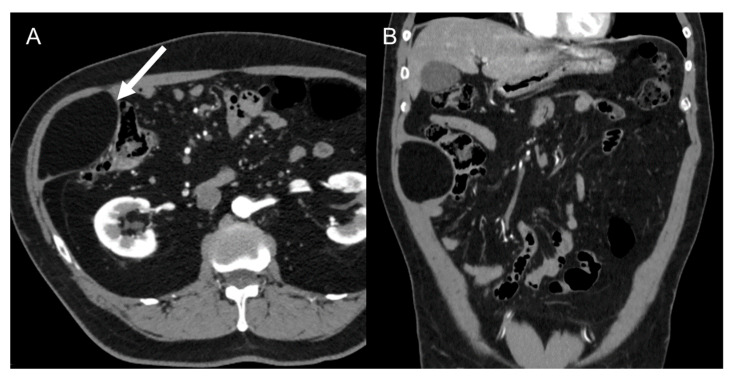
Abdominal lipoma in a 67-year-old man. Axial (A) and coronal (B) contrast-enhanced CT images in the venous phases show a 6.8 cm fatty mass (arrow) in the right abdominal wall, with no internal septa or nodules.
